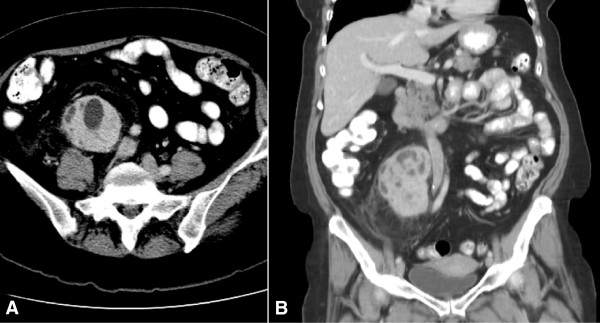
Preoperative imaging. (A) and (B) Axial and coronal computed tomographic scans, respectively, with oral and intravenous contrast showing a large, heterogeneously enhancing retroperitoneal mass deforming the inferior vena cava at the level of the right renal vein (arrow).
